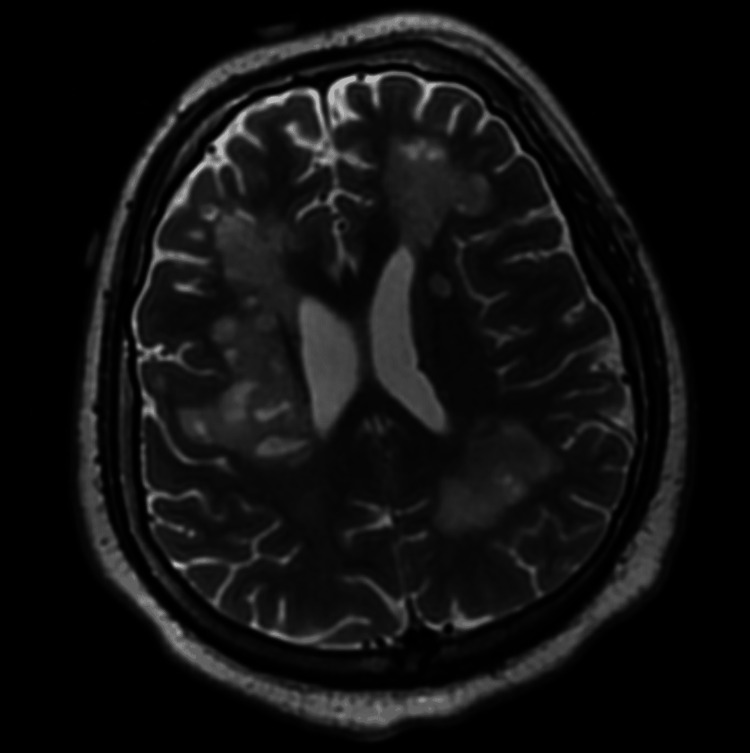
MRI of the brain taken later in the patient’s hospital stay, identifying an increase in the size and quantity of lesions
